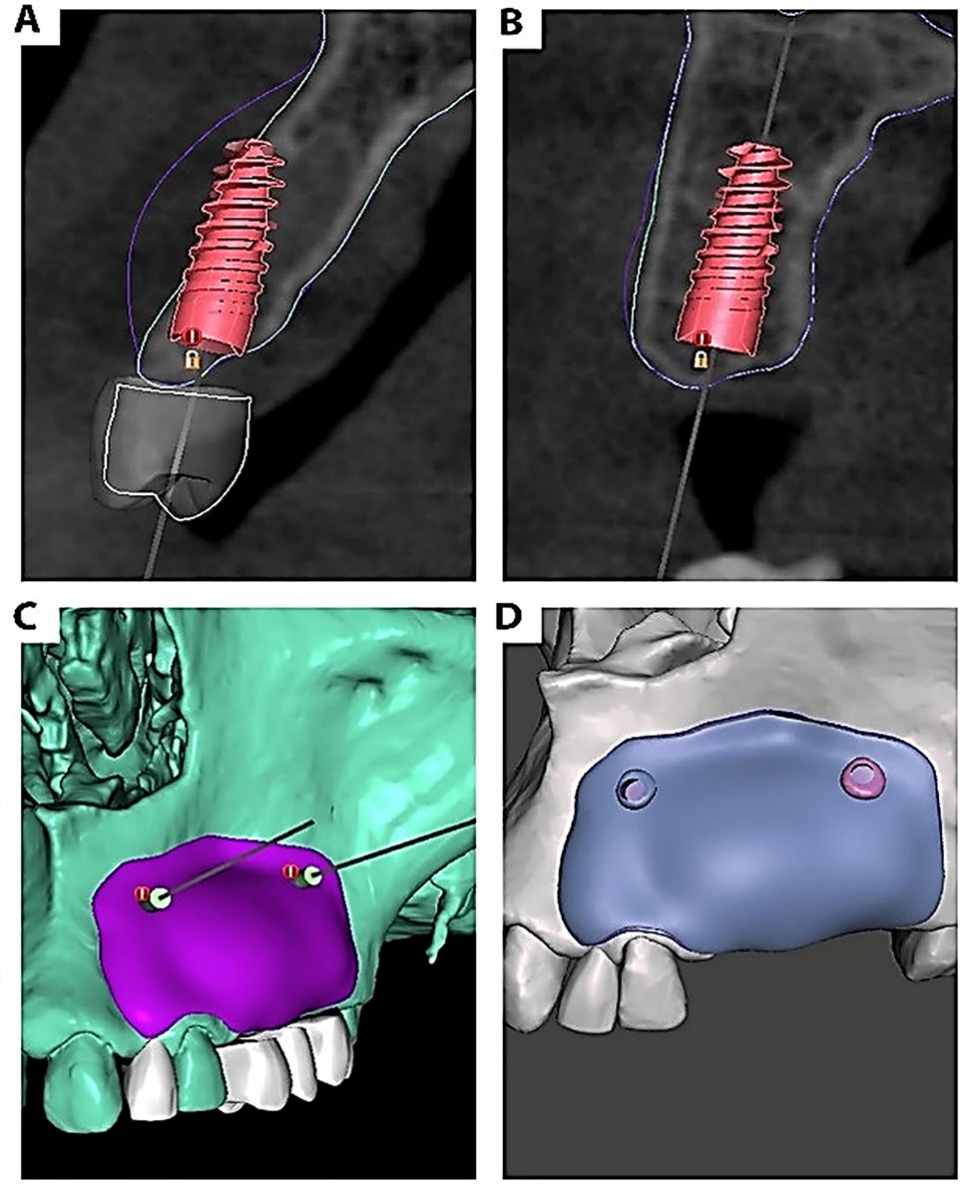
(a) & (b) Screenshots from Blue Sky Plan showing the superimposition of the virtually augmented model (blue outline) over the preoperative radiograph confirming the proper amount of augmentation over the implants (the distal implant (b) is used in this case only as a second reference for linear measurement analysis, as it didn’t need augmentation), (c) a screenshot from Blue Sky Plan showing the planning of the fixation screw positions, (d) a screenshot of Autodesk Meshmixer showing the final design of the membrane over the augmented mode
